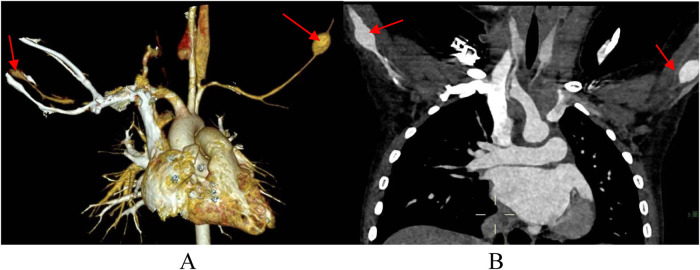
Cardiac CT angiography at 2 years and 8 months of follow-up. (A,B) arrows indicate bilateral brachial aneurysms.
